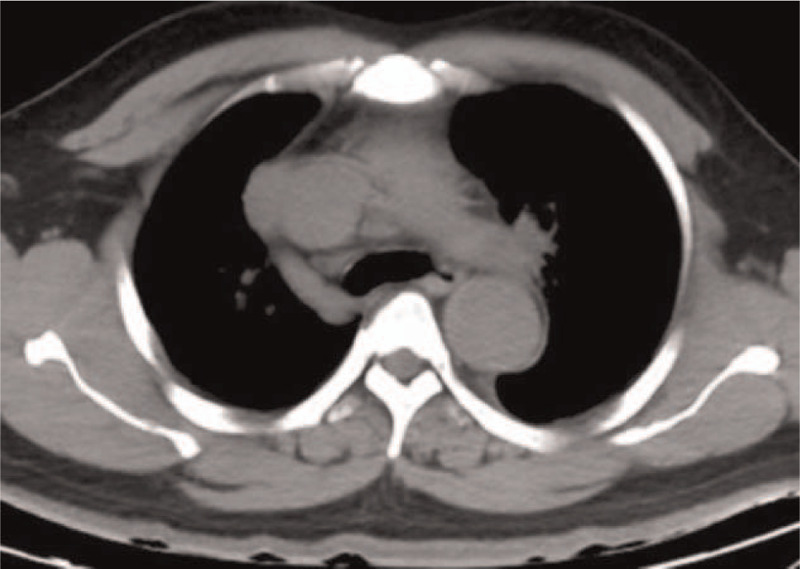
No apparent abnormality is observed at the corresponding layer in the non-enhanced CT image of the same patient as in Fig. 1. CT = computed tomography.
